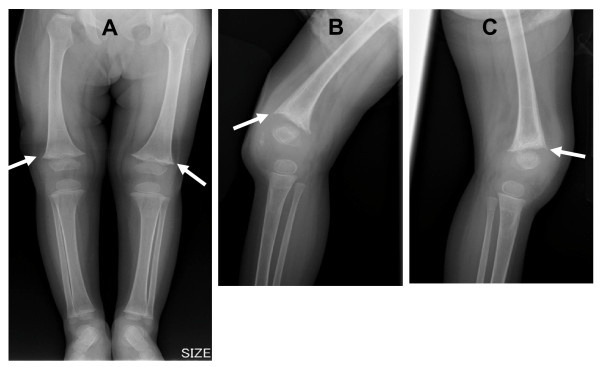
Radiographs at 24 months of age.  (A: frontal view of the lower extremities, B: lateral view of the right knee, C: lateral view of the left knee) show metaphyseal fraying and cupping and widening of the growth plate in the distal femur (arrow).
